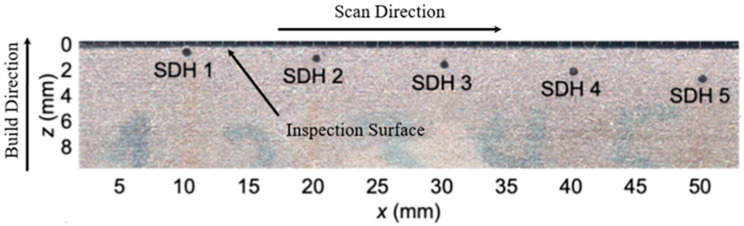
Scaled photographs and geometry of the reference block fabricated via LPBF, including SDHs layout and scan direction used for ultrasonic inspection.
